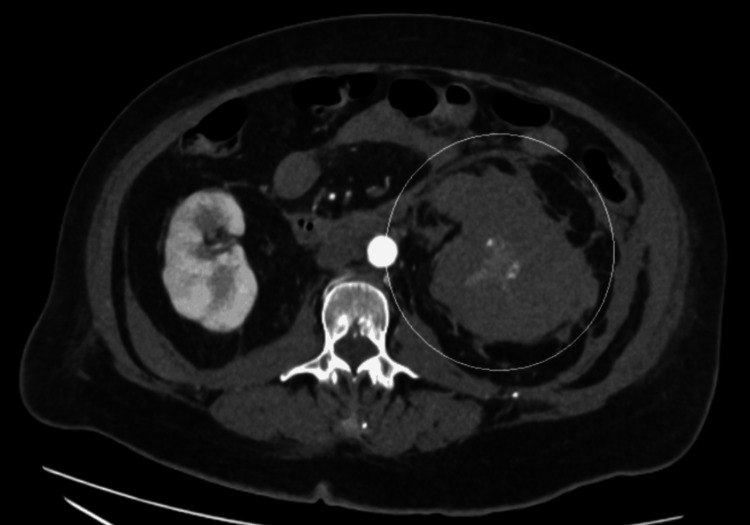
CT angiogram showing increase in the size of left perinephric/subcapsular hematoma
